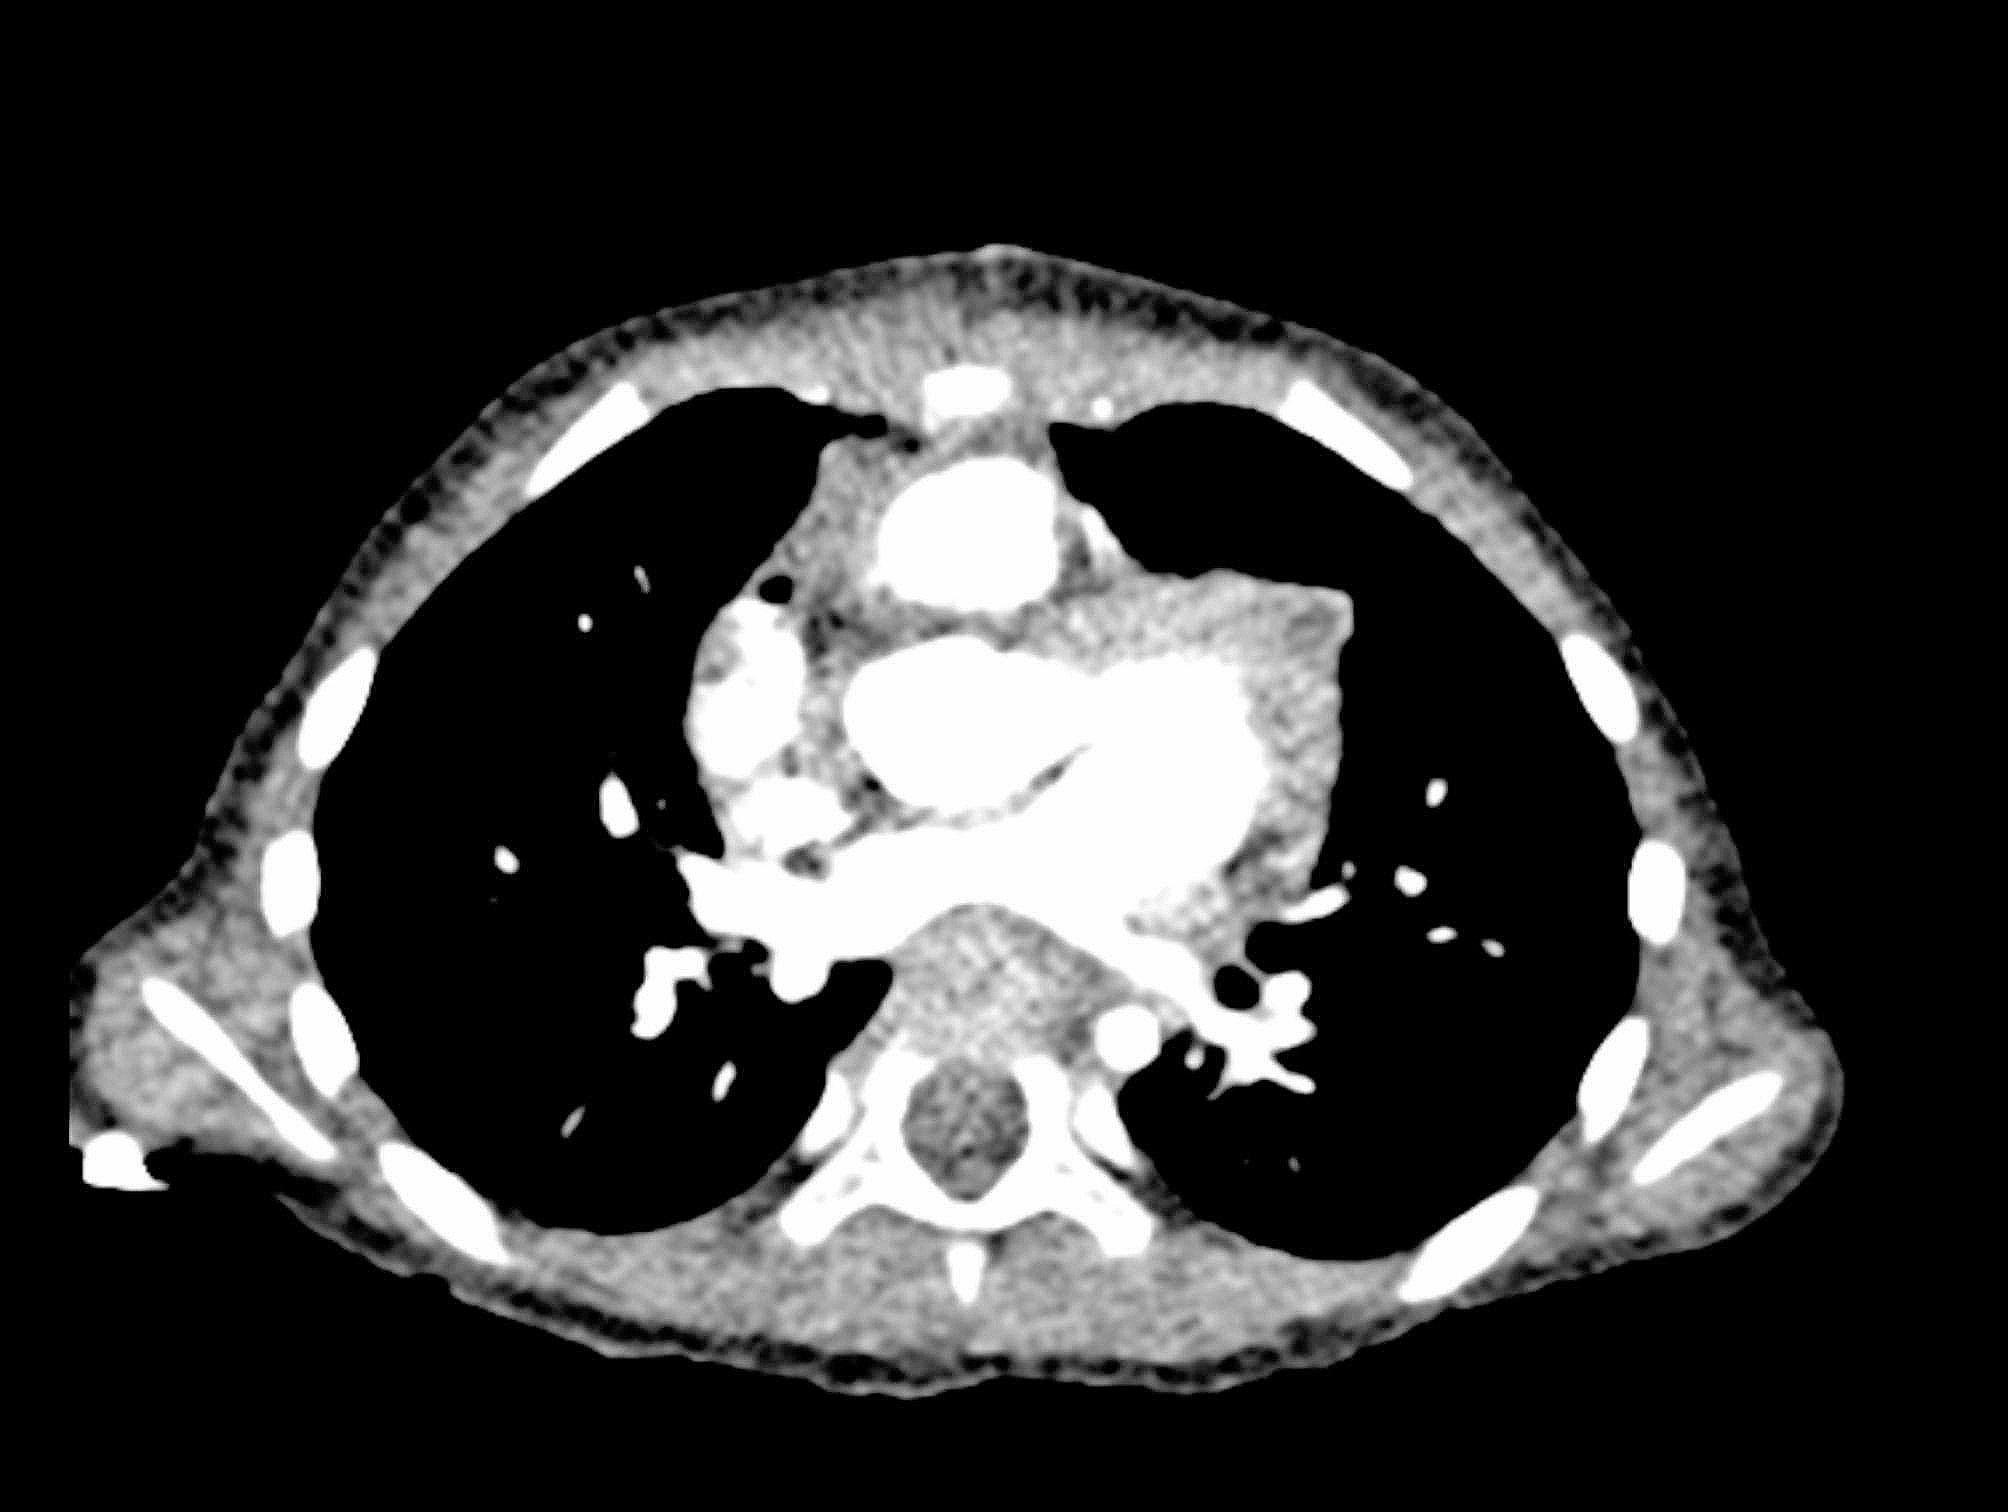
Postoperative computed tomography scan of the pulmonary arteries. Postoperative computed tomography angiography scan: showing patent main, left and right pulmonary arteries. (see supplementary video 1)
